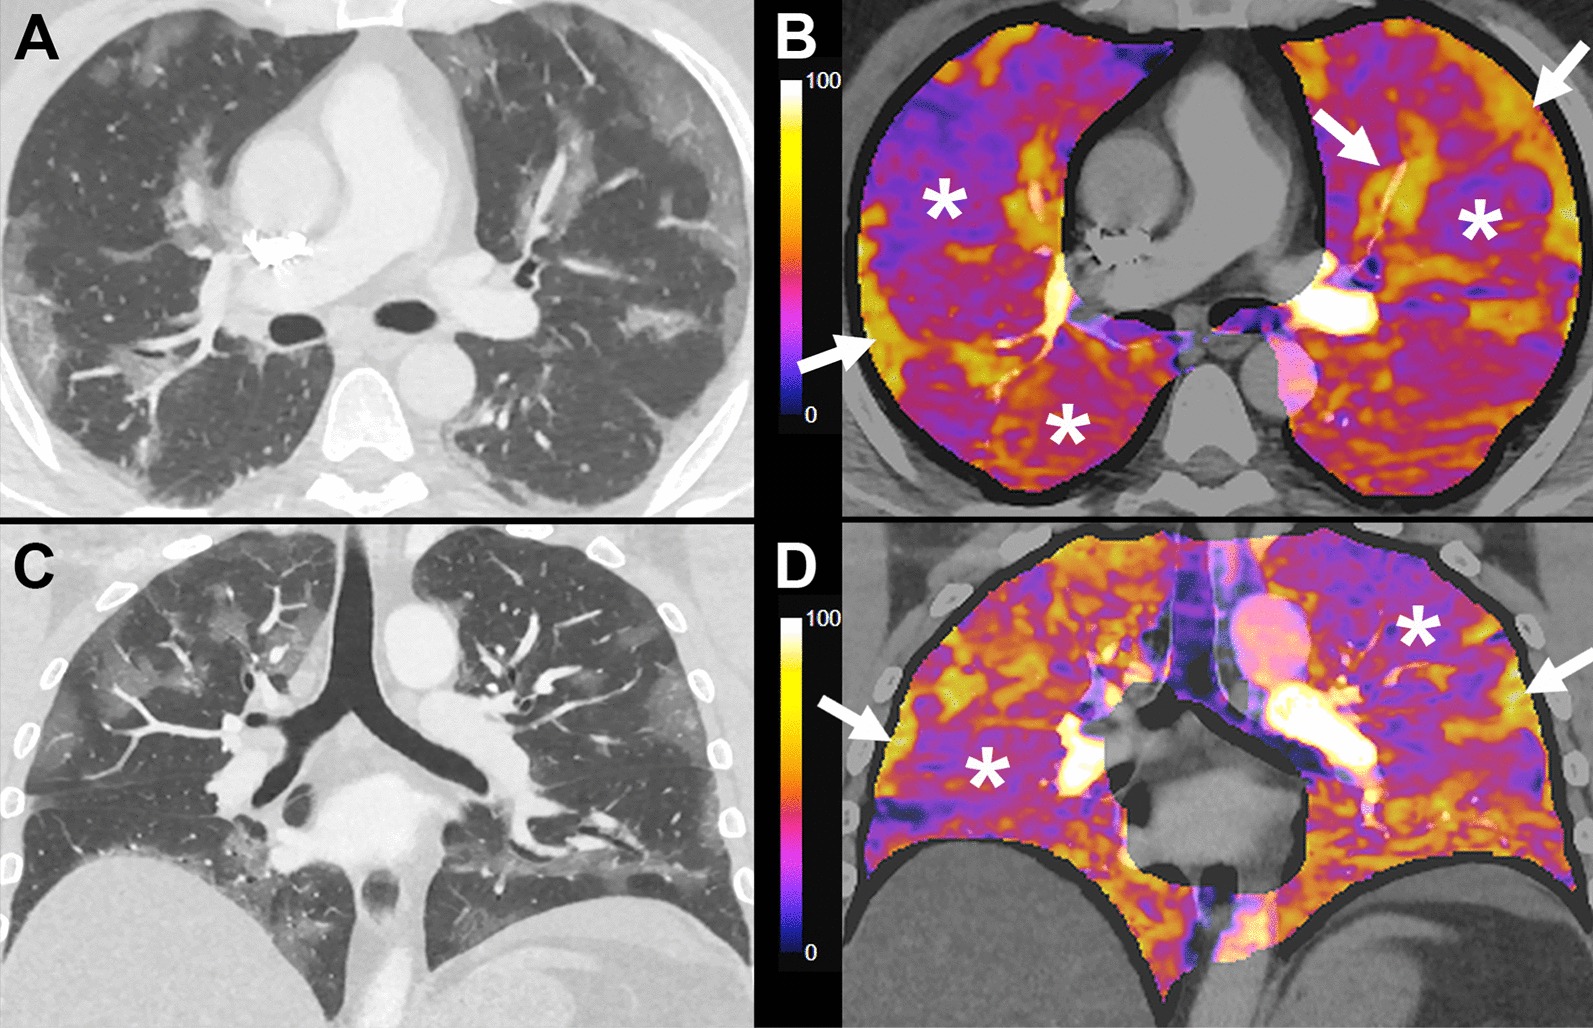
37-year-old male patient, RT-PCR-confirmed COVID-19, 10 days since symptom onset. Admission PaO_2_/FiO_2_ ratio was 240 and d-dimer level was 480 ng/mL. Admitted to the intensive care unit, managed with invasive mechanical ventilation. a, c Axial and coronal lung-window chest CT angiography images show multiple foci of ground-glass opacities, with a predominantly subpleural distribution, with areas of apparently healthy lung parenchyma. Vascular dilatation can be seen in relation to areas of ground-glass opacities. b, d 5 mm reconstruction images of subtraction iodine maps in corresponding axial and coronal planes, show areas of severe hypoperfusion in the corresponding apparently healthy lung parenchyma (*), with increased perfusion in areas of ground-glass opacities (white arrows). This patient evolved in a similar fashion to the L (type 1) phenotype described by Gattinoni et al.
